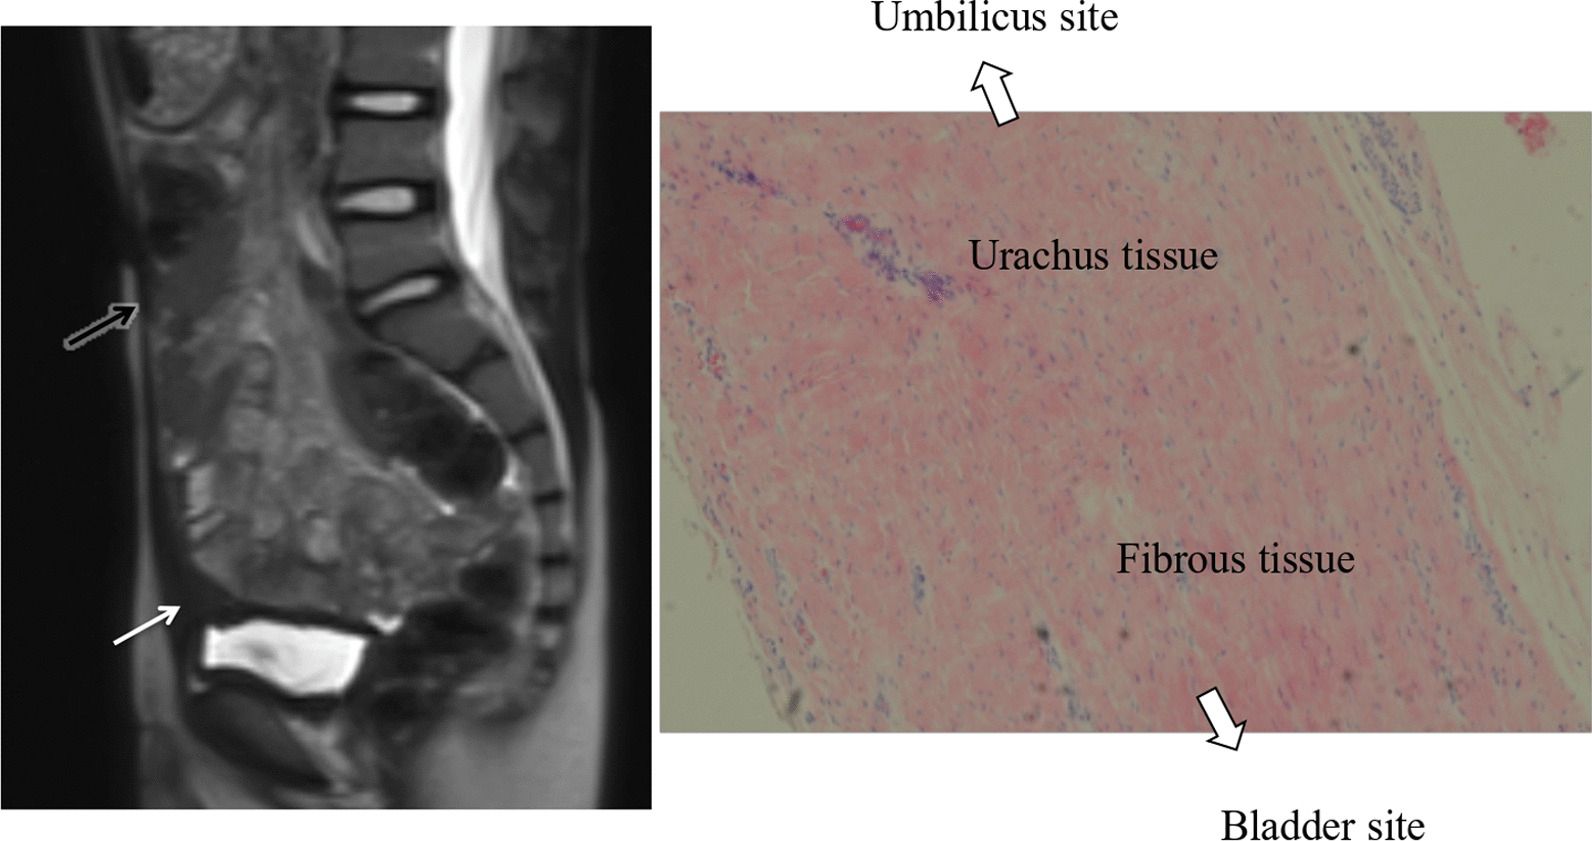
MRI (T2-weighted) shows that there is no urachal remnant at the bladder apex (white arrow). The urachal remnant was resected at the fibromuscular cord-like structure (black arrow). Pathological findings indicated urachus tissue at the umbilicus site and no urachus tissue at the fibromuscular cord-like structure; only fibrous tissue was observed
